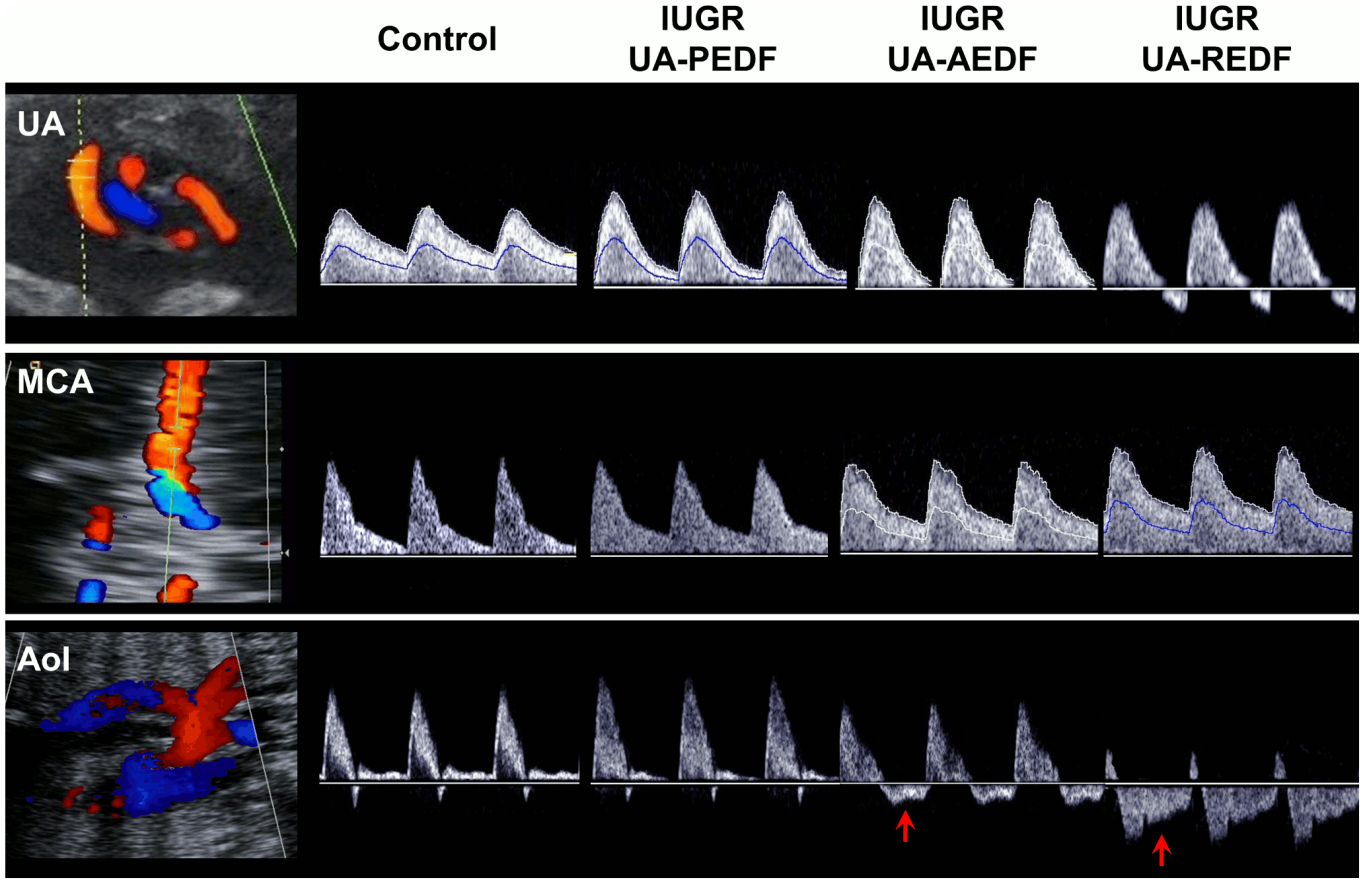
Doppler ultrasound data of the study individuals. Doppler recordings from umbilical artery (UA) (top), middle cerebral artery (MCA) (middle) and aortic isthmus (AoI) (bottom) for the control and the three intrauterine growth restricted (IUGR) fetuses with present (PEDF), absent (AEDF) or reverse (REDF) umbilical artery end-diastolic flow. Red arrows indicated reversal flow in the AoI.
